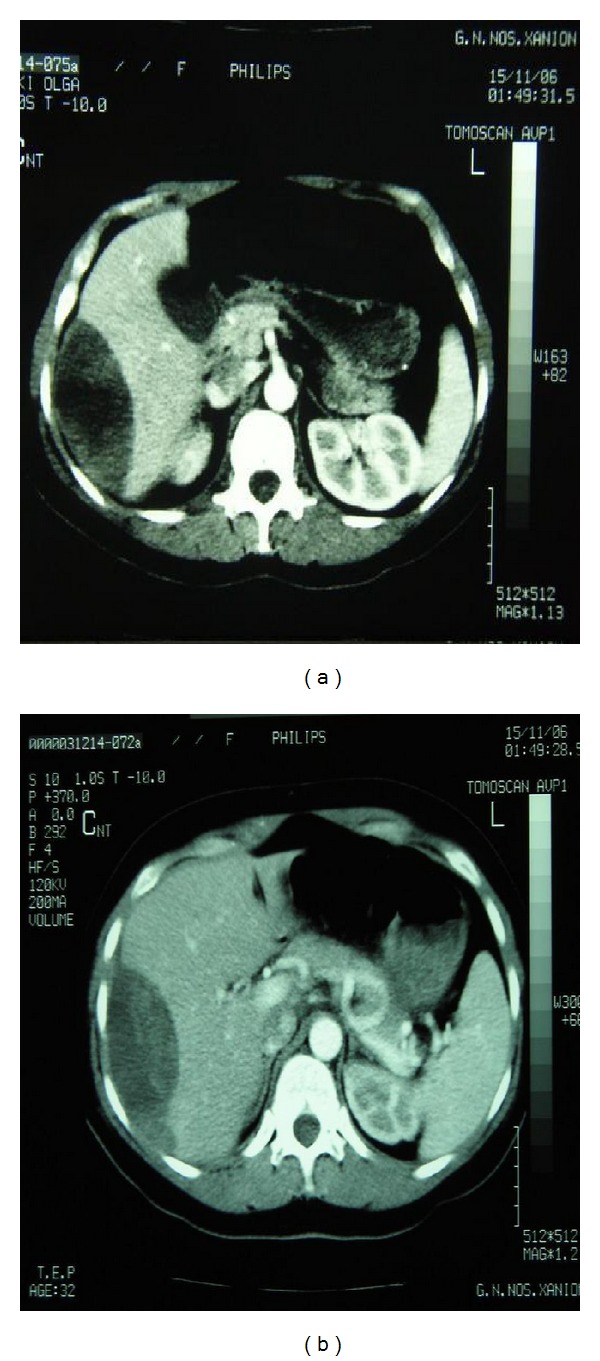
Abdominal computed tomography demonstrating the existence of a large subcapsular hepatic hematoma.
